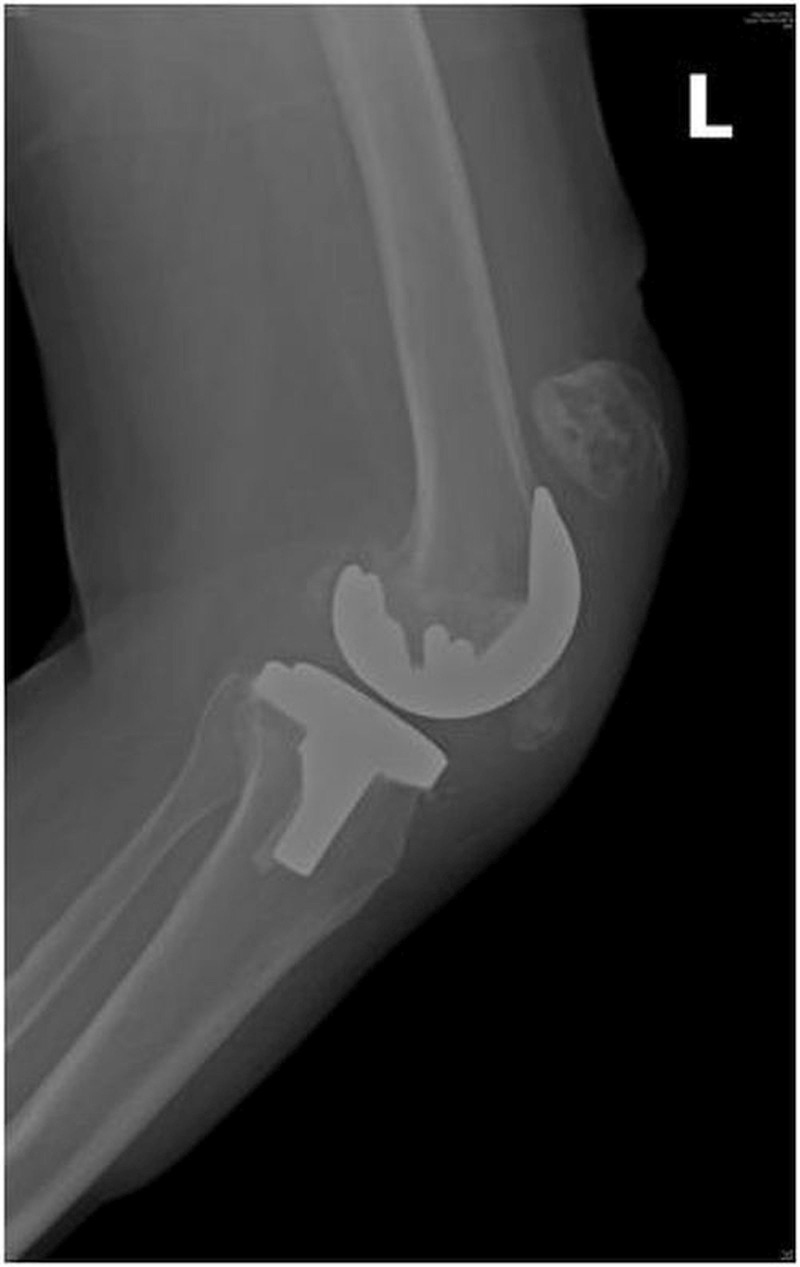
Lateral radiograph of a patient showing a patellar fracture on the left side after TKA
